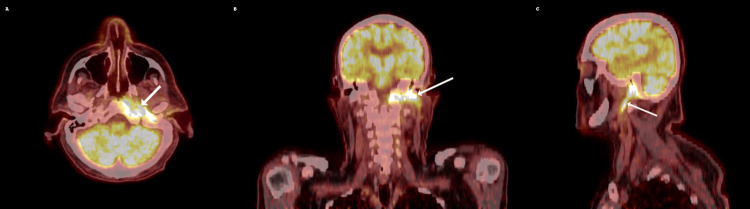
Repeat positron emission tomography (PET) scan 3 months after initial imaging showing stable fluorodeoxyglucose (FDG)-avid lesion (white arrow) involving posterior left nasopharynx, skull base, and tracking along the osseous course of the facial nerve with associated osseous erosion in transverse (A), coronal (B), and sagittal (C) views.
